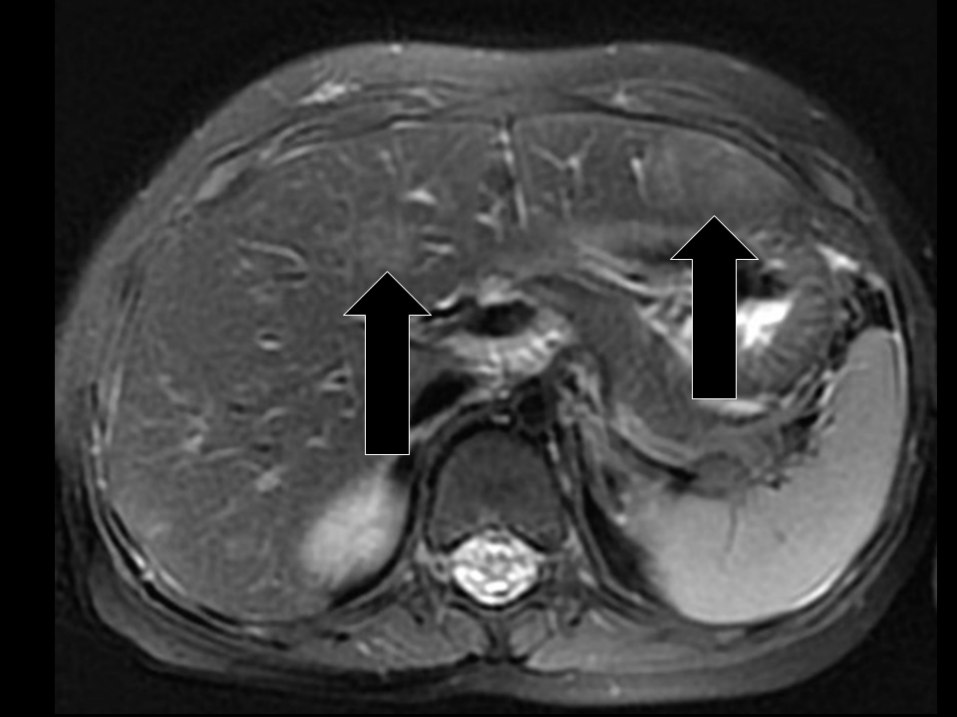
Axial T2-weighted Sequence Obtained as Part of a Dedicated Liver MRI Study to Further Evaluate the Lesions Seen by Ultrasound On this MRI study, a total of seven liver lesions were detected, three of which were not detected by ultrasound and two of which are shown on this image (indicated by arrows). The left liver edge lesion corresponded with the lesion demonstrated in Figure 1.
